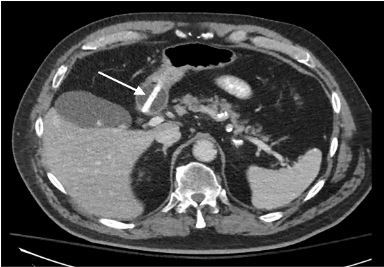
Abdominal computed tomography demonstrating distal portion of replacement gastrostomy tube (arrow) in the pylorus/proximal duodenum.
